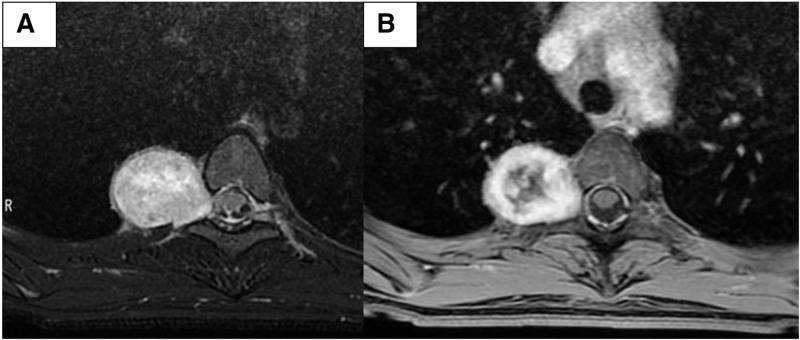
Preoperative magnetic resonance imaging (T2-weighted Image). (A) Pre-contrast-enhanced: a 3.5-cm hyperintense mass was noted at the T3–T4 level. (B) Post-contrast-enhanced: the mass demonstrates heterogeneous enhancement after contrast administration.
